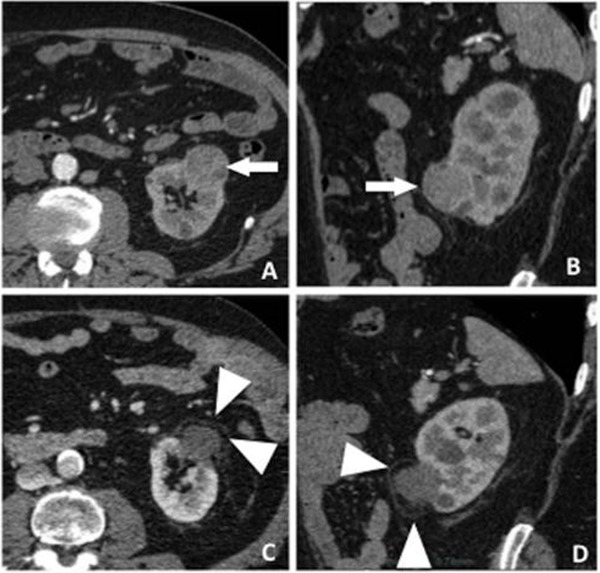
Contrast enhanced, CT scan before cryoablation with axial (A) and coronal (B) reformations show T1a stage exophytic tumor (white arrows). Contrast enhanced, CT scan at 9 months post-cryoablation with axial (C) and coronal (D) reformations show no mass enhancement, confirming complete necrosis of the tumor. The inflammatory rim (white arrowheads) is noted representing the edge of the ablation zone and not residual disease.
